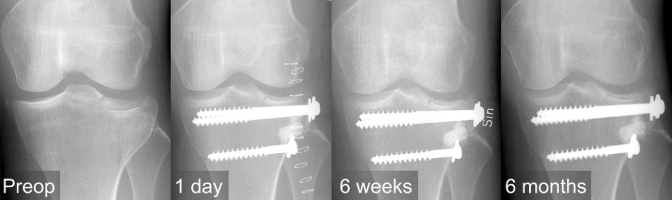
Pure depression fracture (Schatzker III) of the lateral tibial plateau in a 49-year-old woman. Follow-up radiographs showed no loss of reduction.
